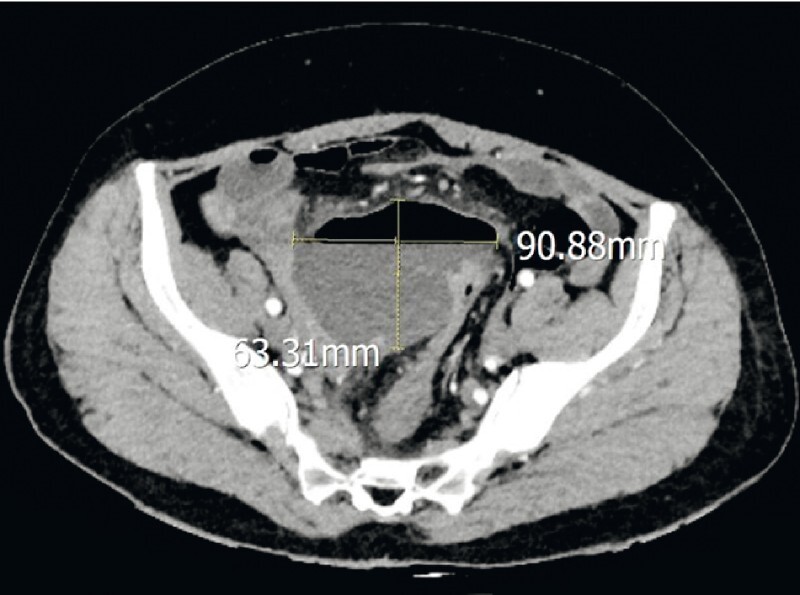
Before endoscopic retrograde appendicitis therapy, computed tomography (CT) showed acute appendicitis with a giant periappendiceal abscess (9.0 cm × 6.3 cm) and intestinal obstruction.
